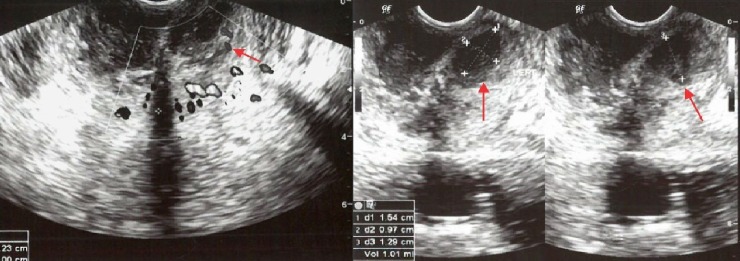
TRUS and power Doppler in a 46-year-old male The image shows multiple irregular hypoechoic nodules (arrow). Color and power Doppler demonsrate increased vascularity. Biopsy revealed inflammatory changes.
